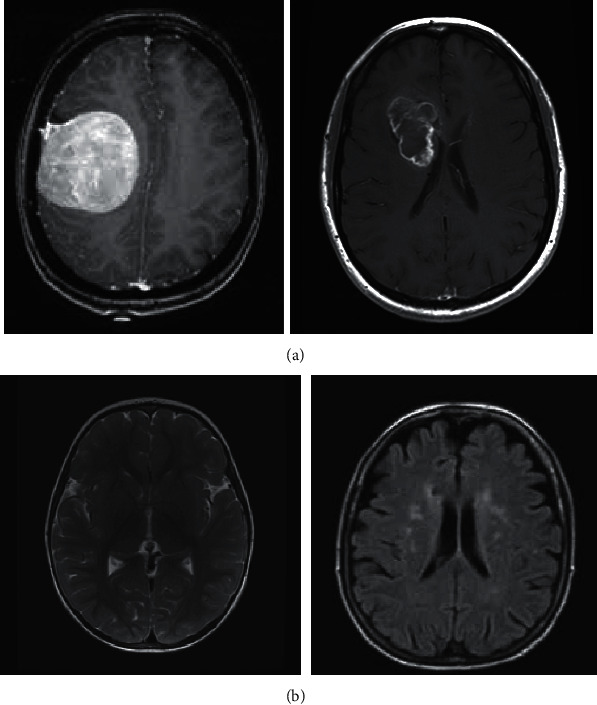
Image of MRI-brain tumor: (a) image of brain tumor patient; (b) nonbrain tumor images.
